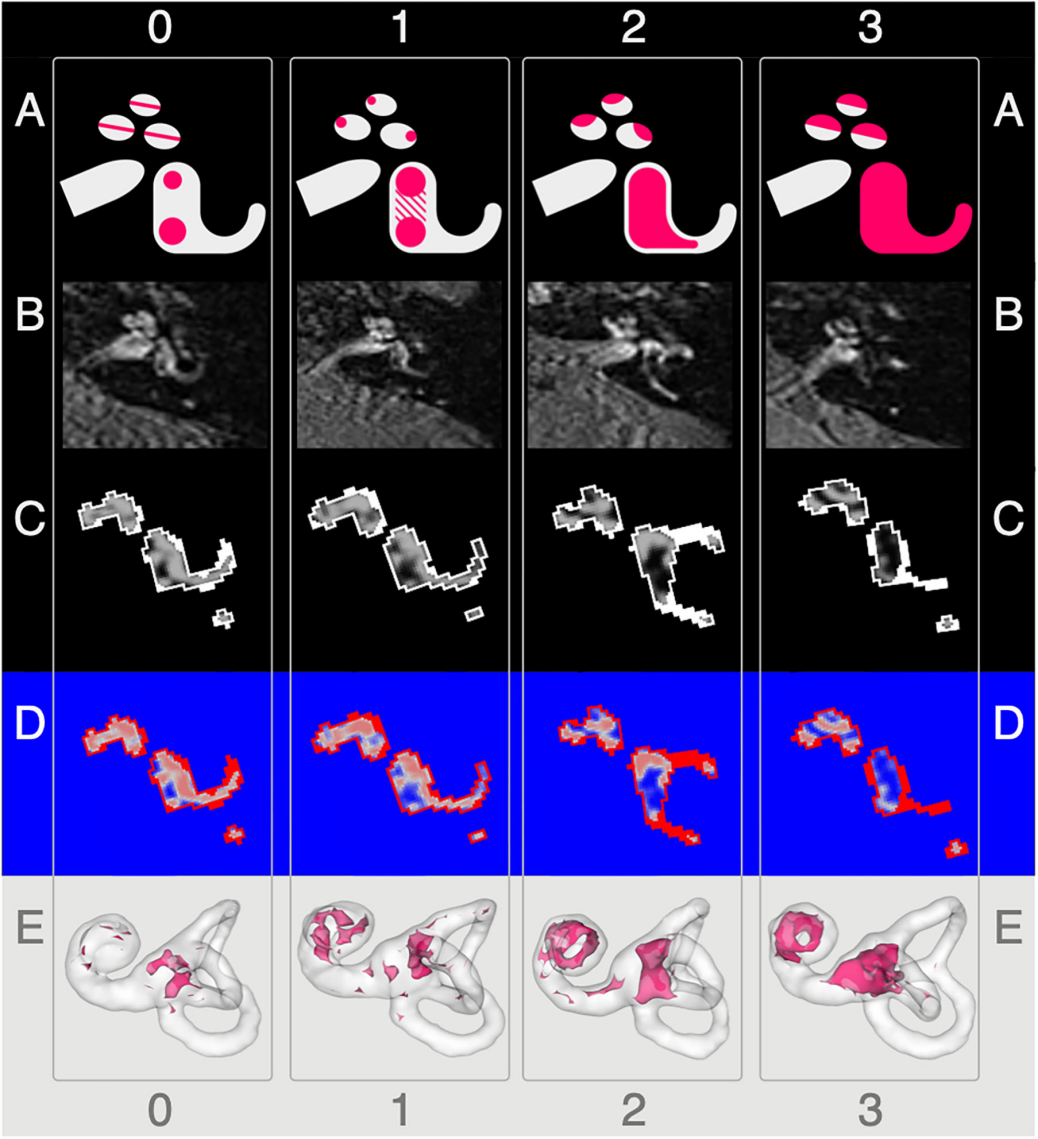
Semi-quantitative (SQ) grading used for the endolymphatic hydrops. The vertical columns show the different semi-quantitative (SQ) grades from 0 to 3 used [cf. Table 1; according to a classification first described in Kirsch et al. (9)]. The horizontal rows each give an overview of how each grade looks (A), in FLAIR raw data (B), after VOLT processing (C), as used for 2D quantification (D), and as used for 3D quantification (E). A detailed description of each grade is given in the second paragraph of subsection ‘Semi-quantitative Grading of the Endolymphatic Space'.
